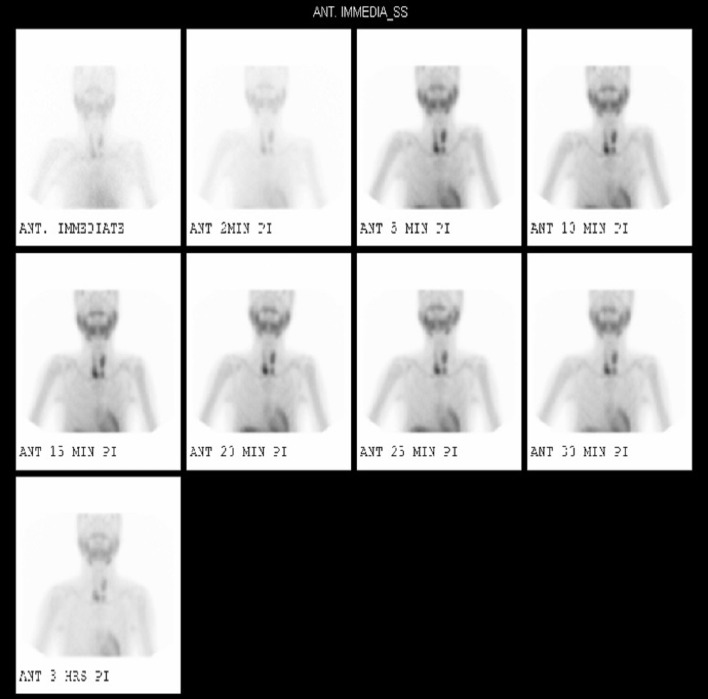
99m TcMIBI (sestamibi) dual-phase parathyroid scintigraphy revealed two foci located below the lower poles of both thyroid lobes with intact thyroid uptake compatible with parathyroid adenomas.
